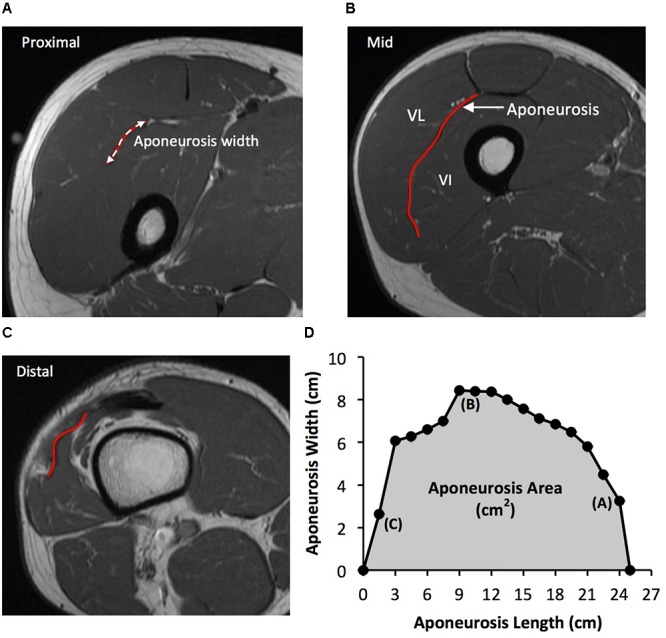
Example axial magnetic resonance images: (A) most proximal, (B) middle, and (C) most distal, showing the transverse length of the vastus lateralis (VL) deep aponeurosis which was traced manually in order to measure aponeurosis width. (D) A cubic spline curve was fitted through the aponeurosis width data points measured at 1.5 cm intervals from the most proximal and distal image where the aponeurosis was visible (aponeurosis length) and the area under the curve was defined as vastus lateralis aponeurosis area.
